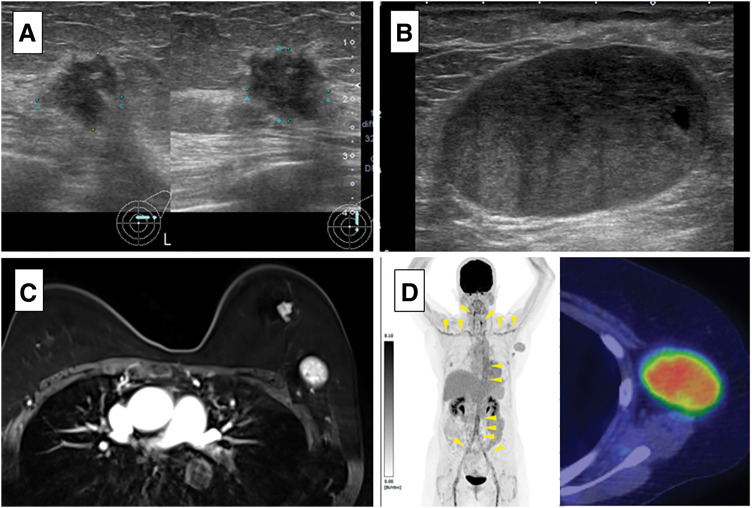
Imaging findings of breast cancer and LVV. (A) Breast ultrasonography showed an irregular hypoechoic mass in the upper outer quadrant of the left breast. (B) Ultrasonography showed an enlarged left axillary lymph node. (C) Contrast-enhanced MRI demonstrated an enhancing mass in the left breast and an enlarged ipsilateral axillary lymph node. (D) FDG PET/CT showed abnormal uptake in the left breast lesion, left axillary lymph node, and multiple large vessels, consistent with LVV (yellow arrowheads). SUVmax values were not available from the archived PET/CT report. FDG, fluorodeoxyglucose; LVV, large-vessel vasculitis
